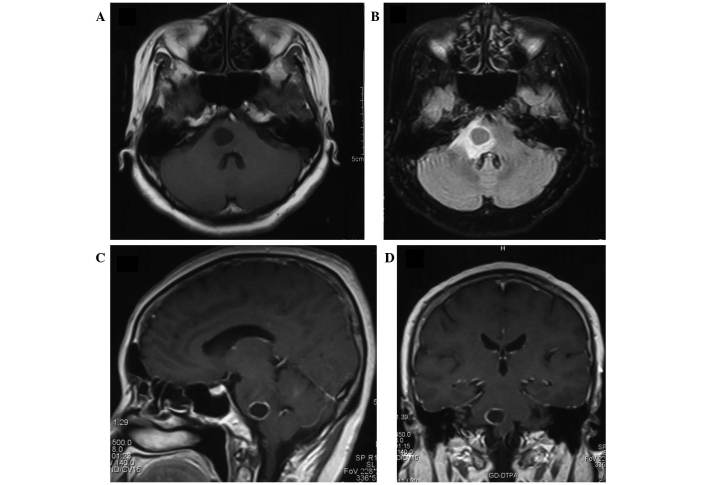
Magnetic resonance images of the cystic brainstem metastasis taken one week after the onset of symptoms. The tumor was 15×12×13 mm in size (volume, 1.3 cm^3^) prior to the initial gamma knife radiosurgery. (A) Axial T1-weighted image; (B) axial T2-weighted, fluid-attenuated inversion recovery image; (C) sagittal contrast-enhanced T1-weighted image; and (D) coronal contrast-enhanced T1-weighted image. FoV, field of view; GD-TPA, gadolinium diethylenetriamine pentaacetic acid.
